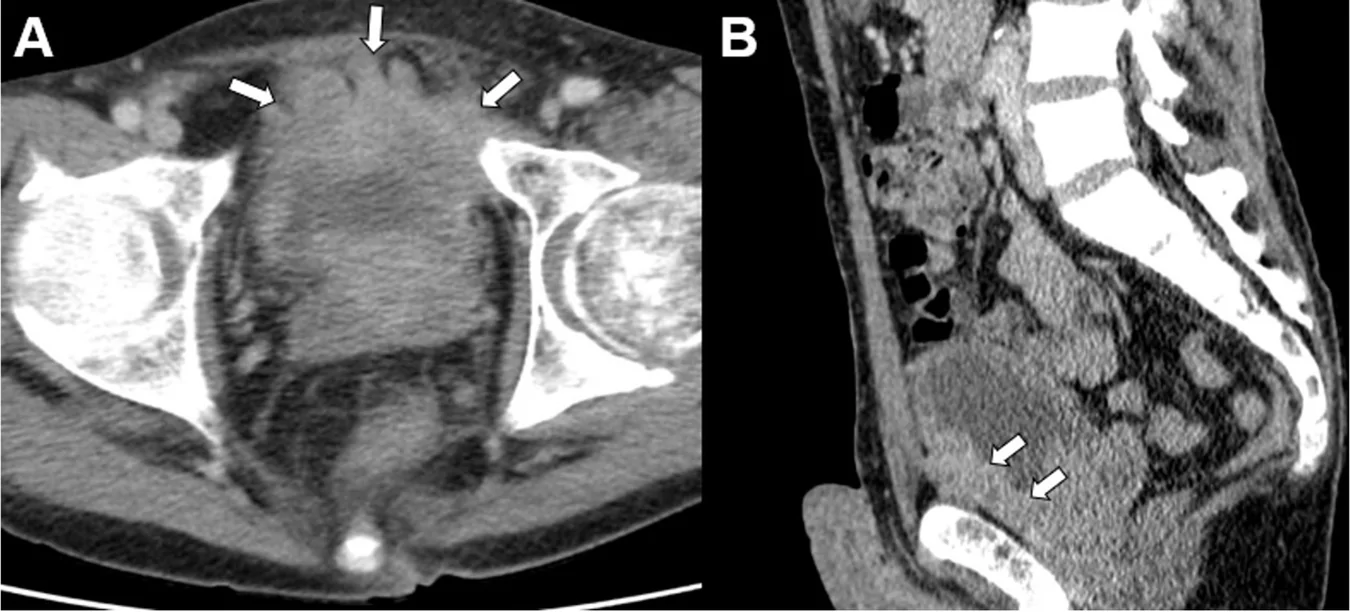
Secondary involvement of the space of Retzius by bladder cancer. Contrast-enhanced axial (A) and sagittal (B) CT images demonstrate tumoral invasion into the space of Retzius (arrows)
